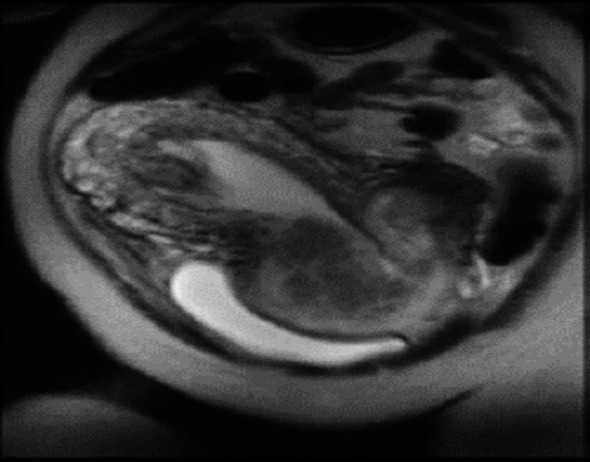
MRI showing bicornuate uterus with placenta increta
